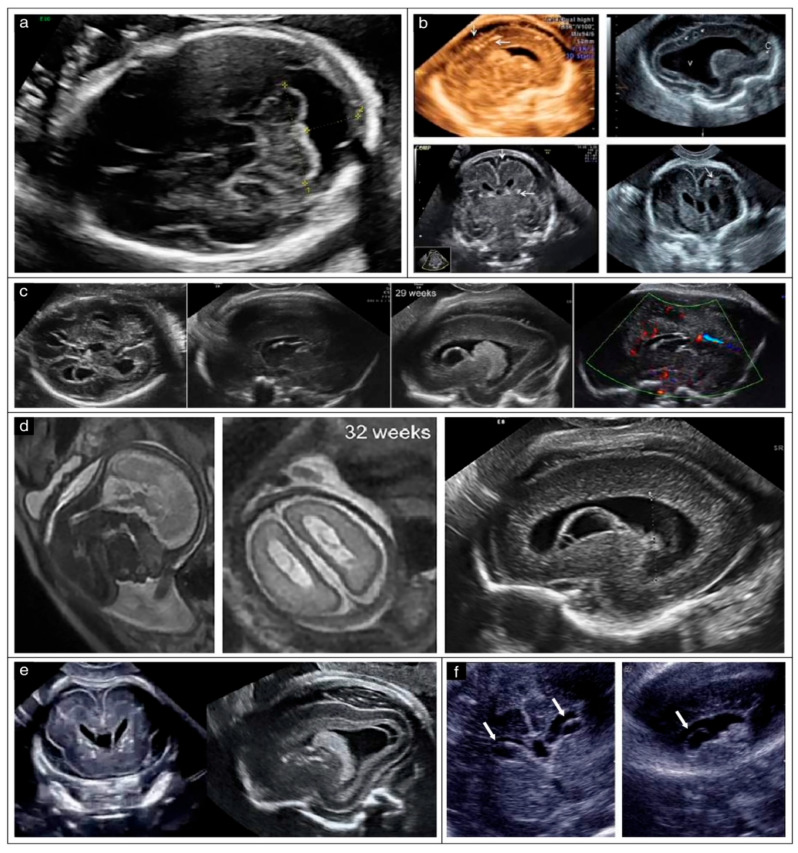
Ultrasonographic cranial features typical of CMV infection [10]. (a) Megacisterna magna, (b) intracranial calcifications, (c,d) ventriculomegaly, germinolytic cysts, agenesis of corpus callosum and intraventricular adhesions, (c,f) periventricular cystic changes, (d) lissencephaly, (e) cerebral calcifications and periventricular cysts, and (f) subependymal cysts.
